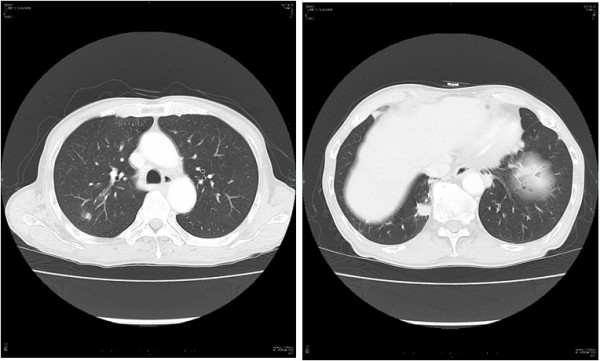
Chest CT view. Chest CT demonstrated multiple nodular densities in both lungs, hilar adenopathy, and a right paravertebral mass lesion.
